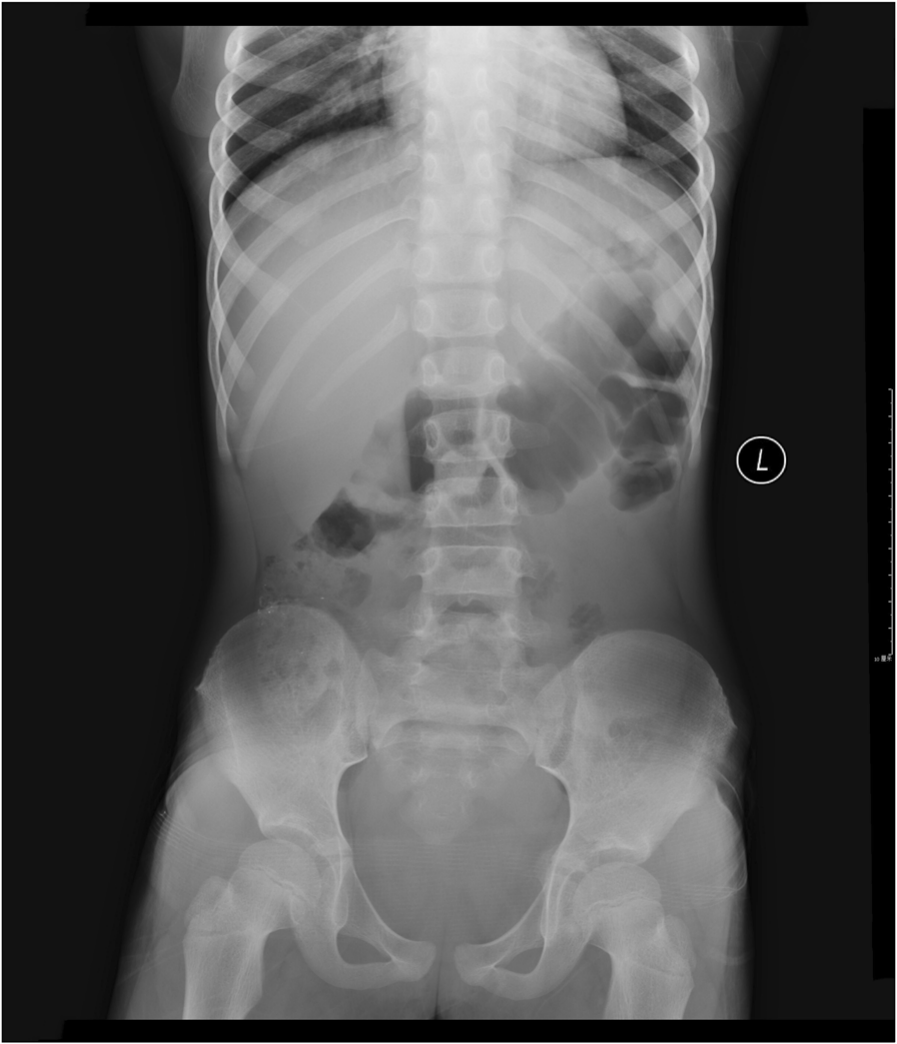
Radiography of the abdomen
